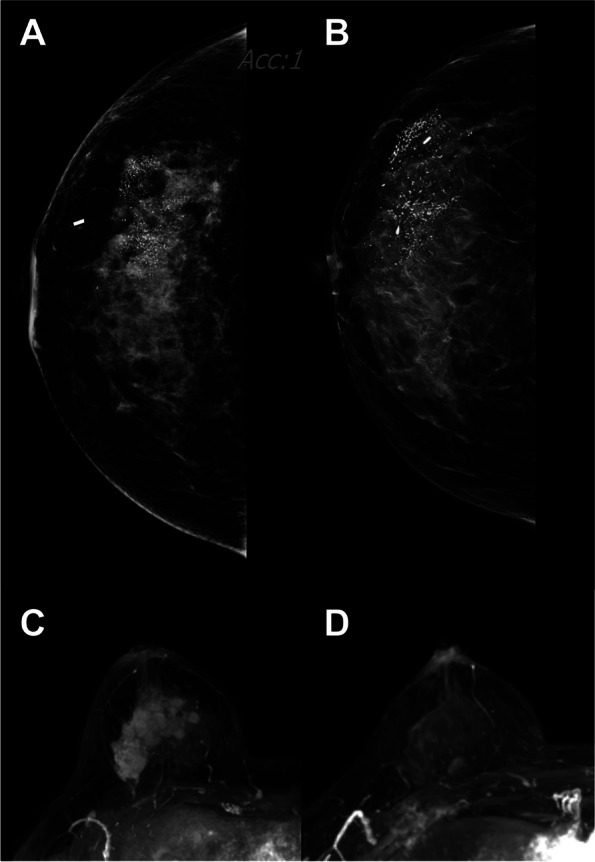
A 44-year-old woman with 53-mm HR^−^/HER2.^+^ subtype in the upper outer quadrant of the right breast. Before NAC, A CC image of mammography showed a 50-mm extent of linear branching microcalcifications. After completion of NAC, B the size and density of the mass were decreased, but the extent of microcalcifications did not decrease on mammography. C A maximum intensity projection (MIP) image from MRI revealed a 55-mm heterogeneous mass with segmental enhancement to the nipple. After NAC, D the MIP image from MRI revealed only small enhancing foci in the upper breast. The patient underwent total mastectomy, and no residual invasive or in situ component was noted. This patient achieved pCR (ypT0)
